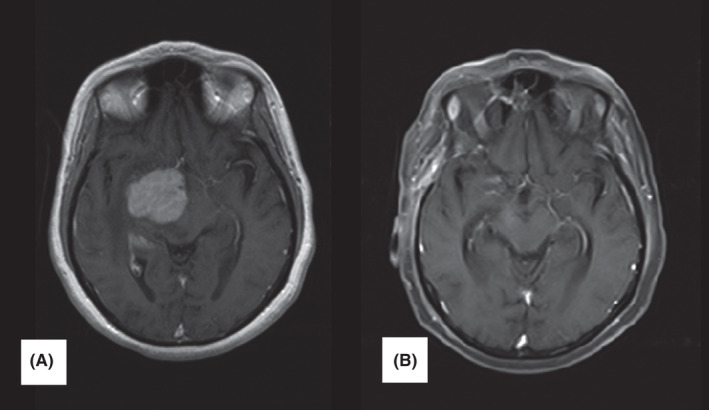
Case number 1. Post‐contrast axial brain MRI. (A) brain MRI before treatment: large intra‐axial mass in the medial right temporal lobe, thalamus, and basal ganglia with avid homogenous contrast enhancement, pressure on the lateral ventricle and midline shift. (B) brain MRI after 20 months follow‐up. An encephalomalacia focus in the right hemisphere without enhancement
